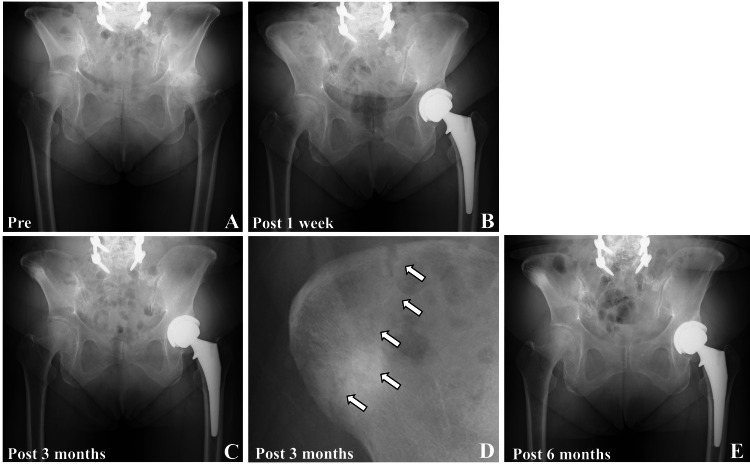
Pre and postoperative X-rays of case two A: Preoperative pelvic X-ray showing left hip osteoarthritis; B: Postoperative X-ray one week after surgery; C: X-ray at three months postoperatively showing a stress fracture of the right iliac crest; D: Enlarged view of the right ilium from panel C. The white arrow indicates the fracture line of the iliac crest. E: The X-ray taken six months postoperatively shows bone union of the iliac crest fracture without displacement.
